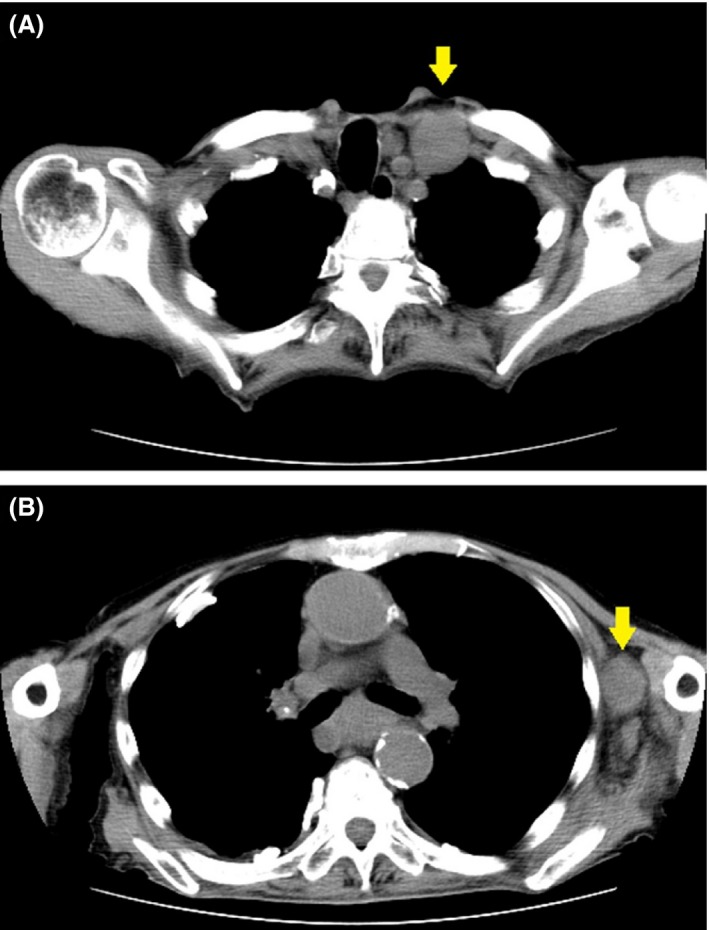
CT imaging revealed a left supraclavicular lymph node metastasis (A, yellow arrow) and a left axillary lymph node metastasis (B, yellow arrow).
